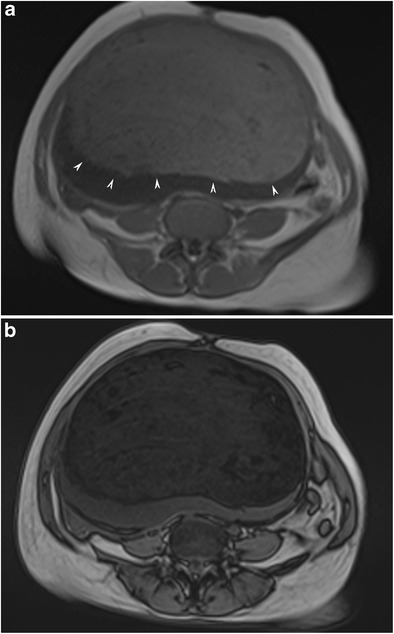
Axial T1-weighted in-phase MR image of a 56-year-old female with mature cystic teratoma (a) shows a large mass with a high signal intensity part creating an interface (arrowheads) with a low signal intensity part. T1-weighted opposed-phase image (b) demonstrates the fat component with decreased signal intensity in the supernatant layer regarding the presence of sebum
